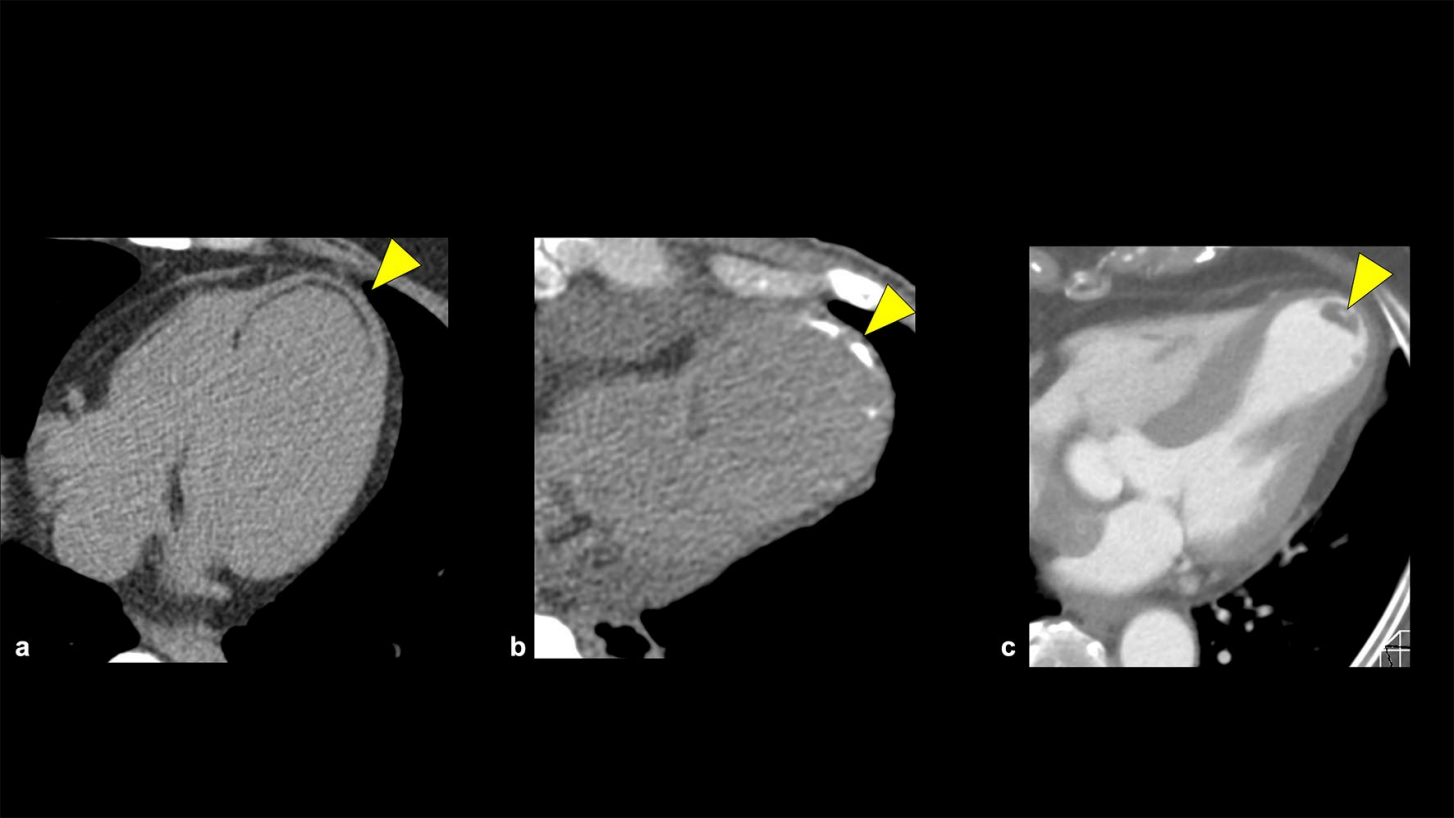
Old myocardial infarction. Old myocardial infarction image shows various CT findings such as fatty degeneration (a) and calcification (b). Patients with old myocardial infarction have a high risk of intracardiac thrombus (c). *CT* computed tomography
